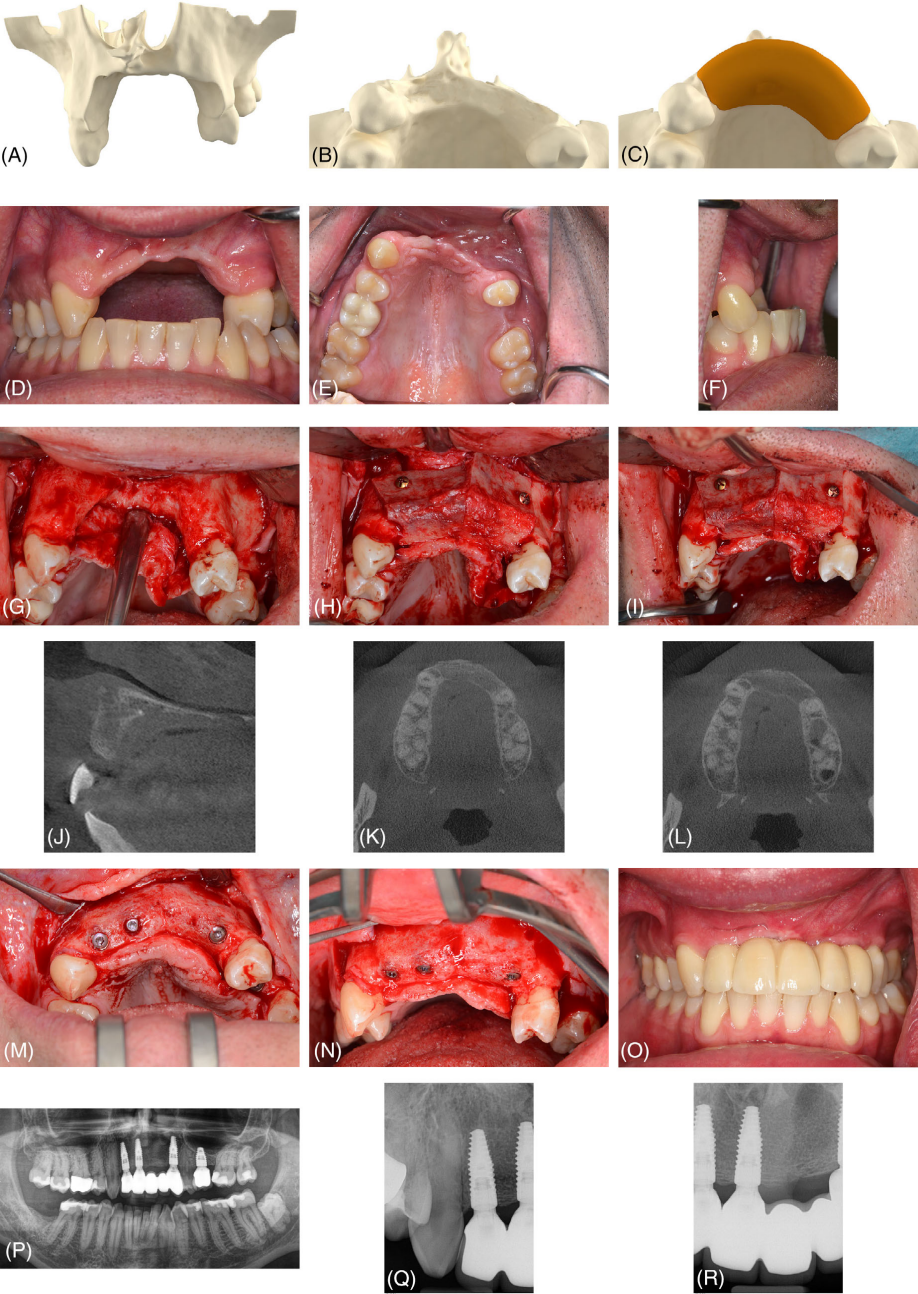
(A, B) Three‐dimensional reconstruction of the radiographic situation prior to bone augmentation; (C) determined alveolar ridge dimension; (D–F) clinical situation showing horizontal and vertical bone defect; loss of alveolar ridge contour and projection (G) intraoperative view of the defect; (H, I) situation after iliac crest block fixation with osteosynthesis screws. The cortical portions of the graft are used to reconstruct the original bone contour and the projection of the maxilla. (J–L) computed tomography images after 3 months of graft healing showing reconstructed interarch relationship (M, N) Intraoperative view at implant placement after 3 months of graft healing. The contour was fully reconstructed, and resorption was minimal. Three implants were left for submerged healing. (O) Clinical situation at follow‐up appointment, with stable soft tissue situation. Superstructure without the need to replace missing tissue with pink porcelain. (P–R) Radiographic follow‐up with stable marginal bone levels.
